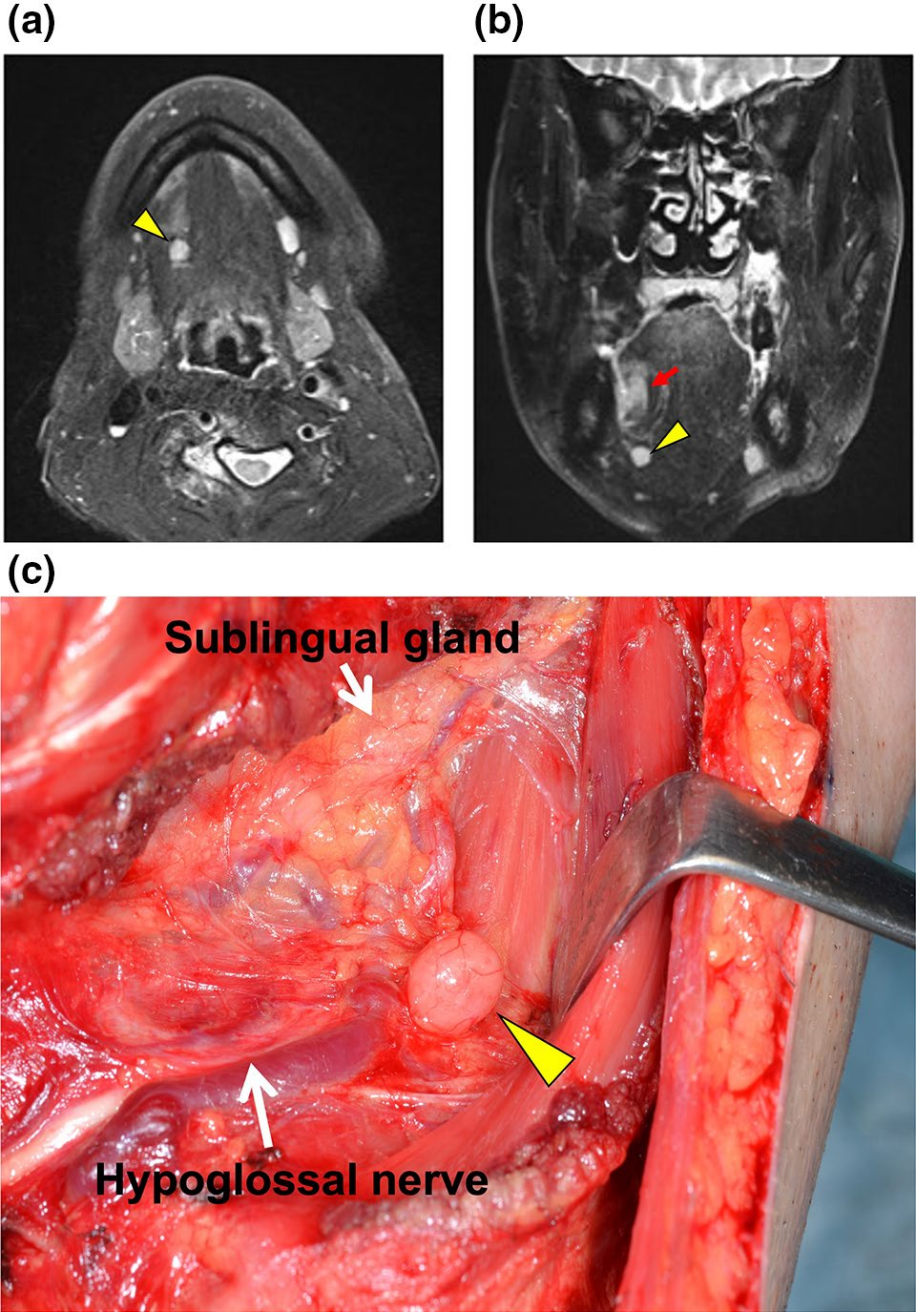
Imaging of lateral lingual lymph node metastasis (LLNM) in the sublingual space. Lateral LLNM (arrowhead) is revealed in the sublingual space on the right side on (a) axial T2-weighted magnetic resonance (MR) and (b) coronal T2-weighted MR images. This metastatic node is independent of the primary tongue tumor (arrow). (c) Lateral LLNM (arrowhead) is located in the proximity of the sublingual gland. The mylohyoid muscle is retracted anteriorly.
